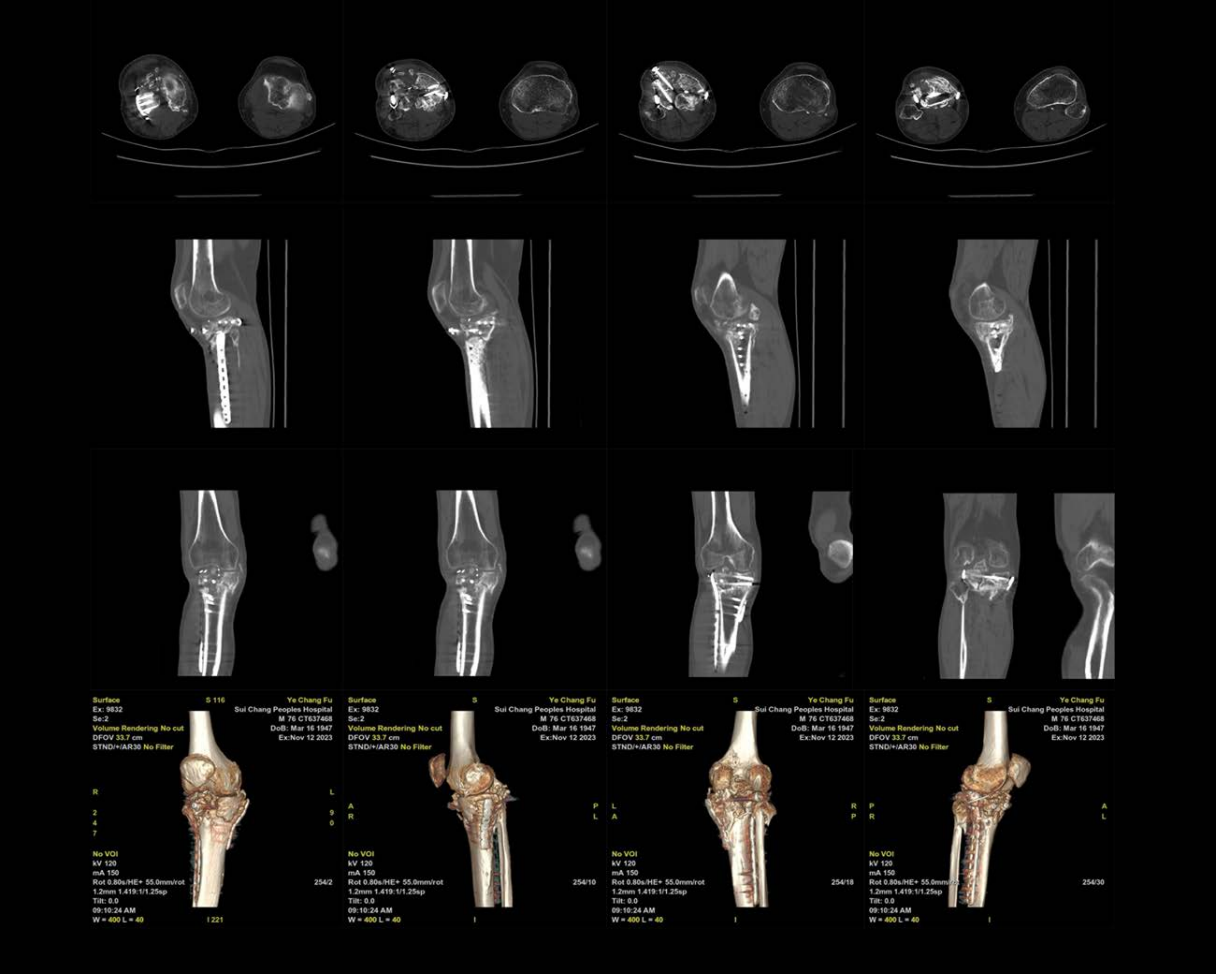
Computerized tomography revealed revealed nonunion of the TPF in this patient (Fig. 7; date of examination: November 12, 2023). Axial (A; B; C; D), agittal (E; F; G; H), coronal (I; J; K; L), and 3-dimensional (M; N; O; P) CT.
